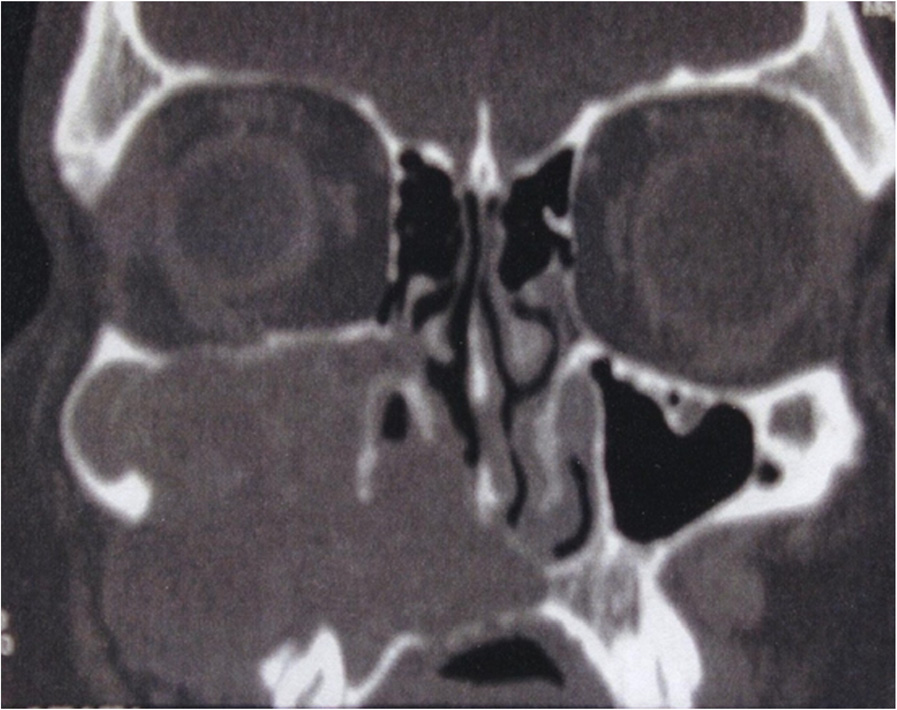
Pre-operative computed tomography scan. Image shows a solid neoformation occupying the right maxillary sinus, erosion of the anterior and posterior walls of the sinus and mass extension to the corresponding right dental roots and the nasal cavity, with compression and deflection of the nasal septum. There was no enhancement after contrast administration.
